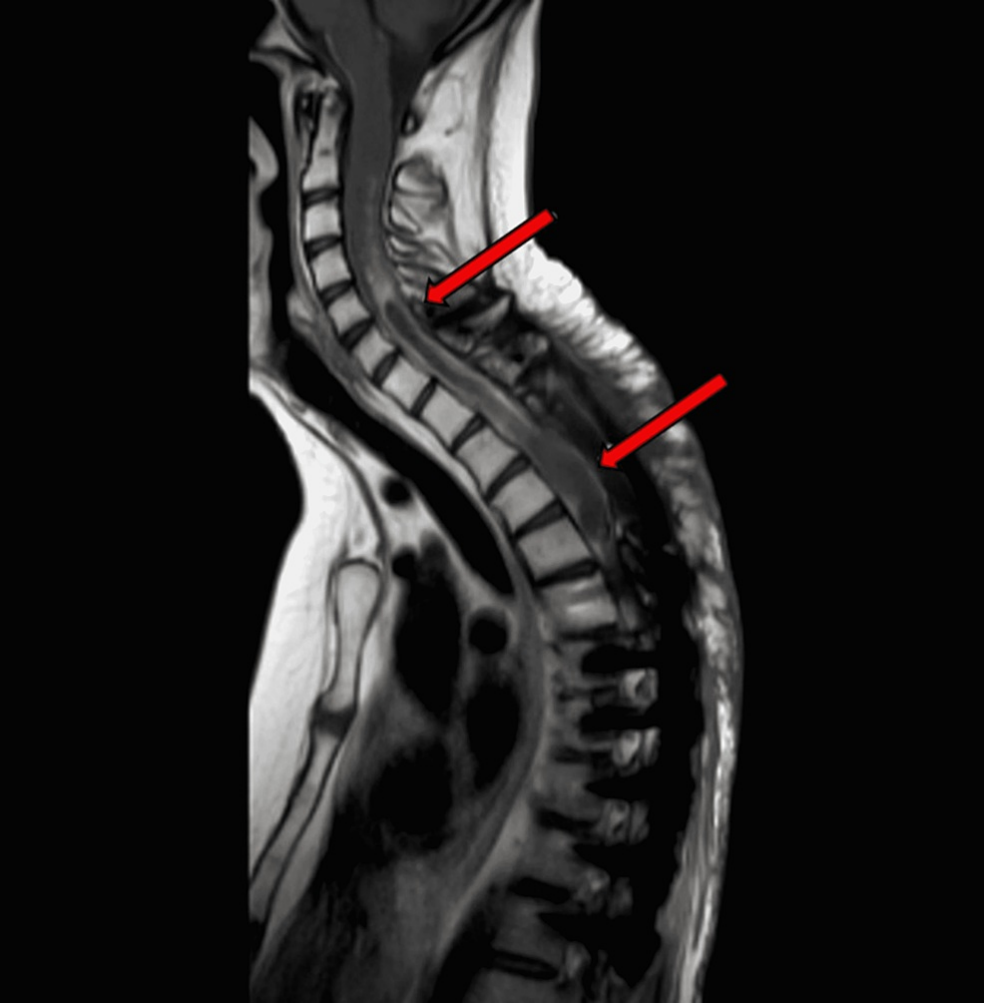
Sagittal MRI of thoracic spine showing decreased signal within the parenchyma of the upper cervical and thoracic cord (arrows)
